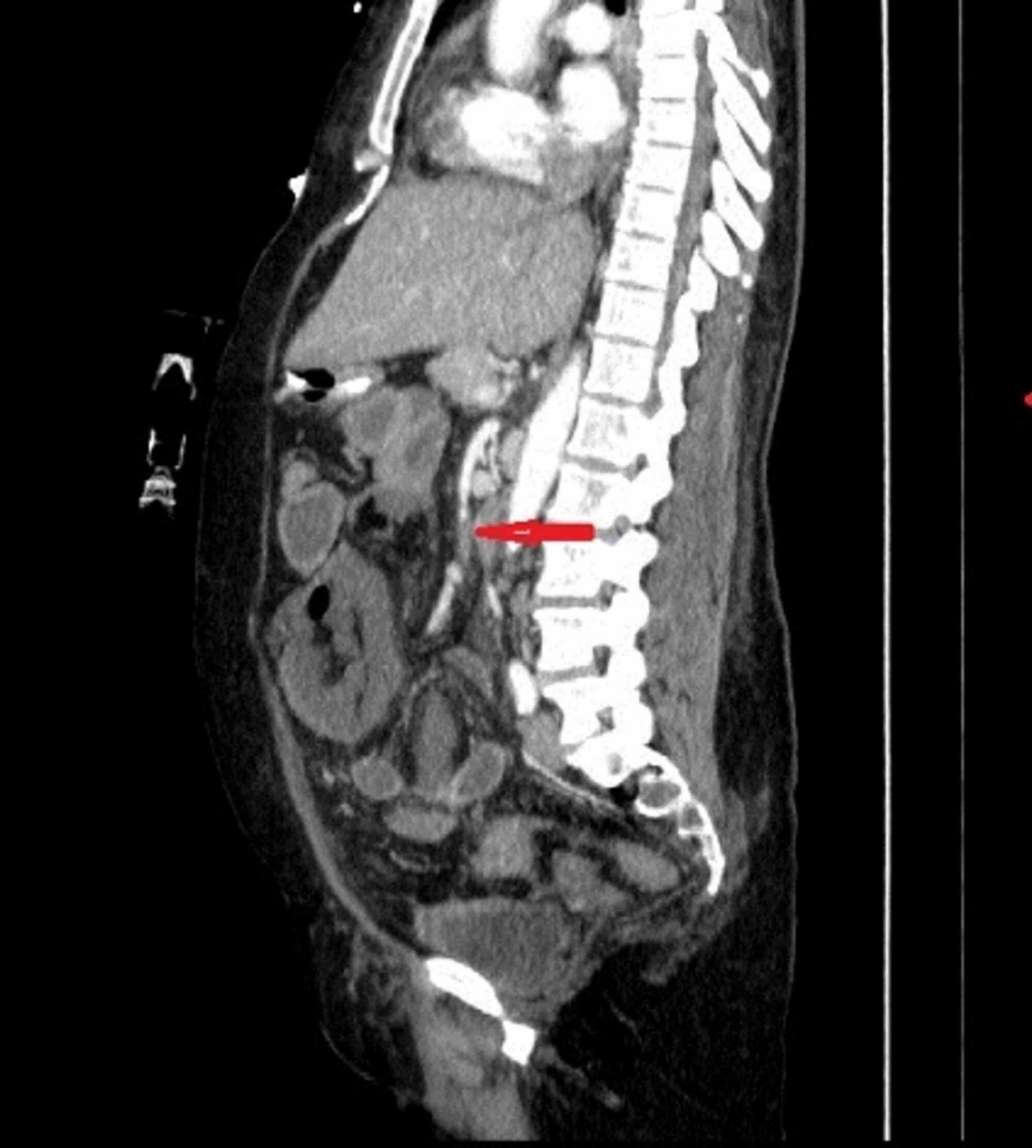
CT scan sagittal view: upper mesenteric artery thrombosis (arrow)
